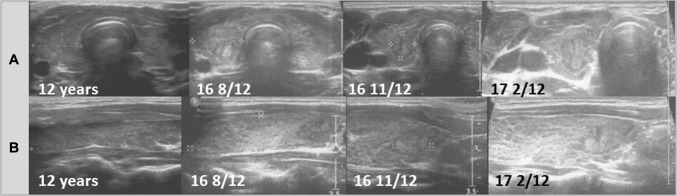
Patient 1. Transversal (a) and longitudinal (b) scans of the hypoechogenic thyroid gland revealed formation of the nodule 5 years since referral and 4 8/12 years since last ultrasound assessment. The growth of the nodule was fast from 8.1 × 9.5 × 13.1 mm to 10.8 × 10.4 × 16.4 mm in 6 months (82% increase). During follow-up, the echogenicity of the thyroid gland increased
